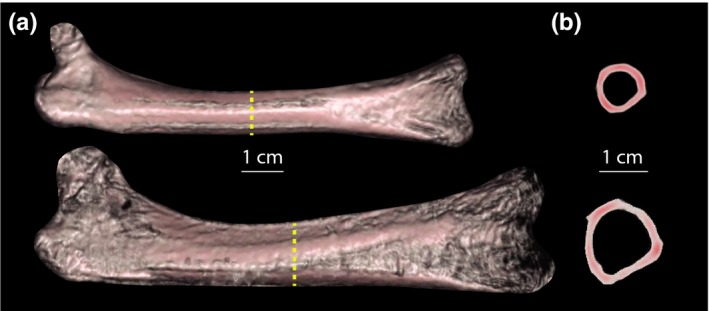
Femur length and cross‐sectional area for 14‐week‐old wild and domestic turkeys, from CT scans. (a) The wild turkey femur is from individual #48 (top) who weighed 3.49 kg and had a femur length of 10.6 cm. The domestic turkey femur (bottom) is from individual #8, who weighed 10.17 kg and had a femur length of 12.5 cm. The CT scans are left aligned to show relative lengths. The bone in the epiphyses of the domestic turkey femur appears to be less dense, as indicated by the holes near the distal ends of the bone. (b) Femur cross sections of the bones depicted in A, from a midpoint along the length of the bones (midshaft), approximated by the yellow dashed line. The domestic turkey femur is <20% longer than the wild turkey femur, but nearly twice the diameter
